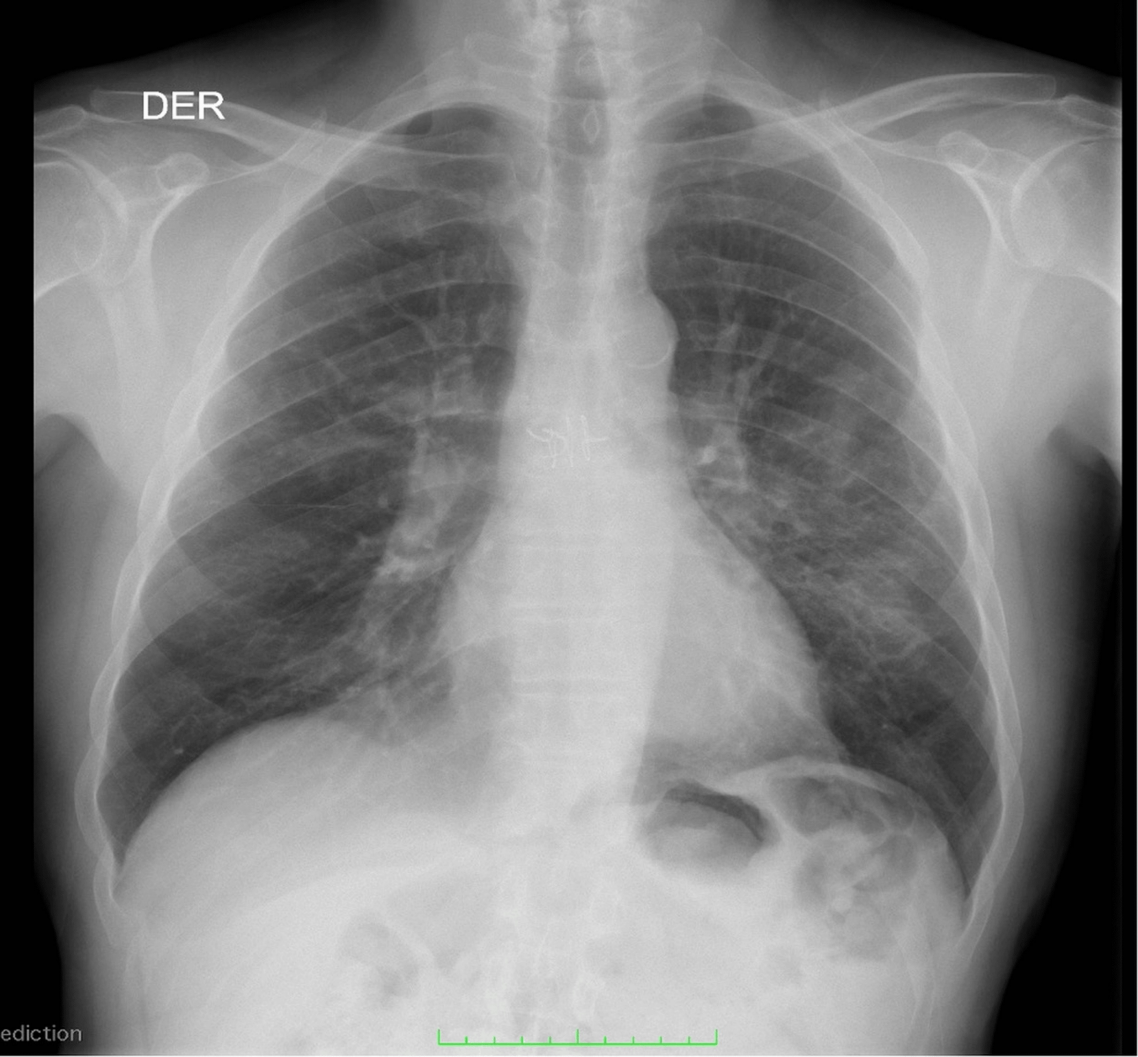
Admission chest radiograph
